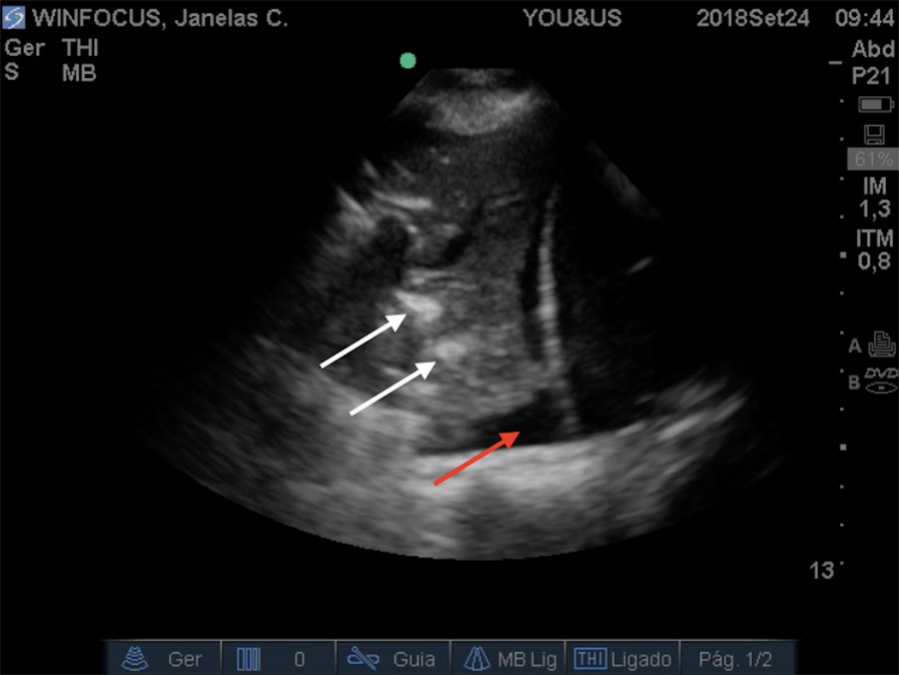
Sonographic features of lung consolidation. The echo-texture of lung becomes similar to the liver. White arrows indicate air bronchograms. Red arrow indicates pleural effusion
